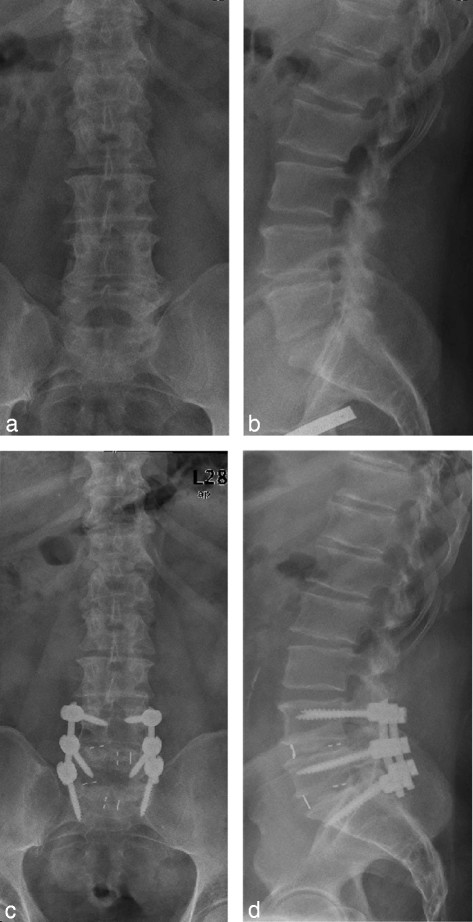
A 56-year-old man with degenerative disease at levels L4/5 and L5/S1. He benefited after probatory infiltration. One year after surgical treatment with two-level decompression and TLIF L4/5 and L5/S1, there were no changes in the measured score levels of chronicity, depression, activities of daily living and pain intensity compared to the preoperative/pre multidisciplinary pain program treatment scores. a, b) Preoperative x-ray, a.p. and lateral view. c, d) One year after surgery.
